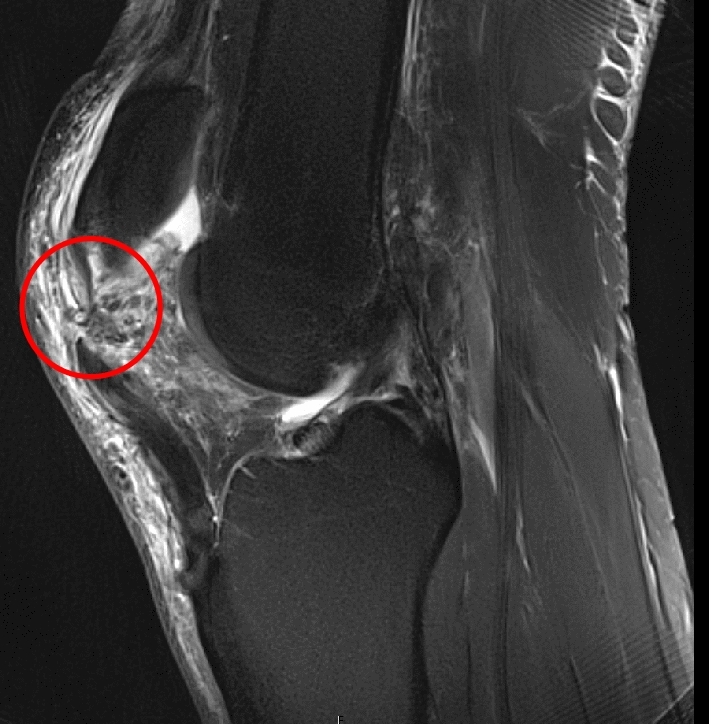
Intermediate-weighted (proton density) sagittal magnetic resonance imaging showing proximal avulsion of the patellar tendon (red circle)
